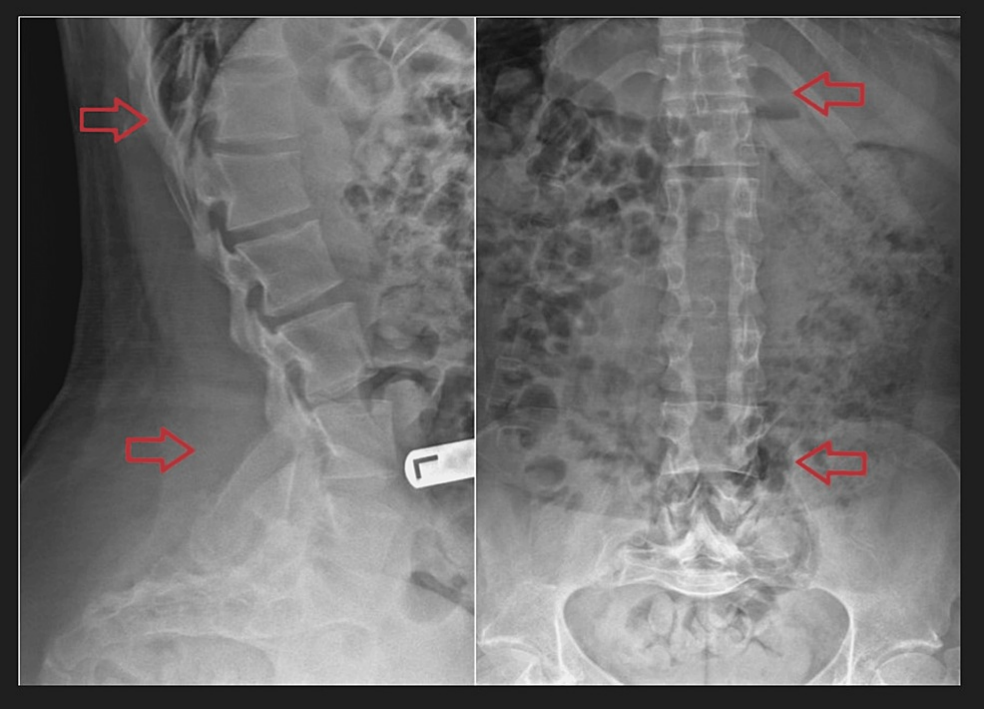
Postoperative X-ray following T12-L5 laminectomy with bilateral partial facetectomy. Left image (lateral view): red arrows indicating theterminal vertebrae (T12/L5) of the involved laminectomy. Right image (anteroposterior view): red arrows indicating theterminal vertebrae (T12/L5) of the involved laminectomy.
